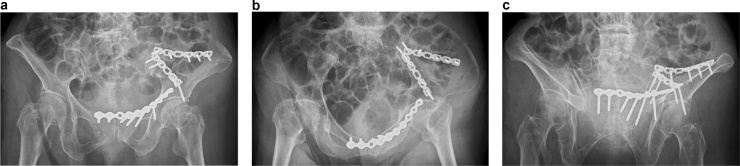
Representative post-operative X-ray images taken following surgical repair of fragility fracture of the pelvic ring. (a) Post-operative pelvic anteroposterior view. (b) Post-operative pelvic inlet view. (c) Post-operative pelvic outlet view.
